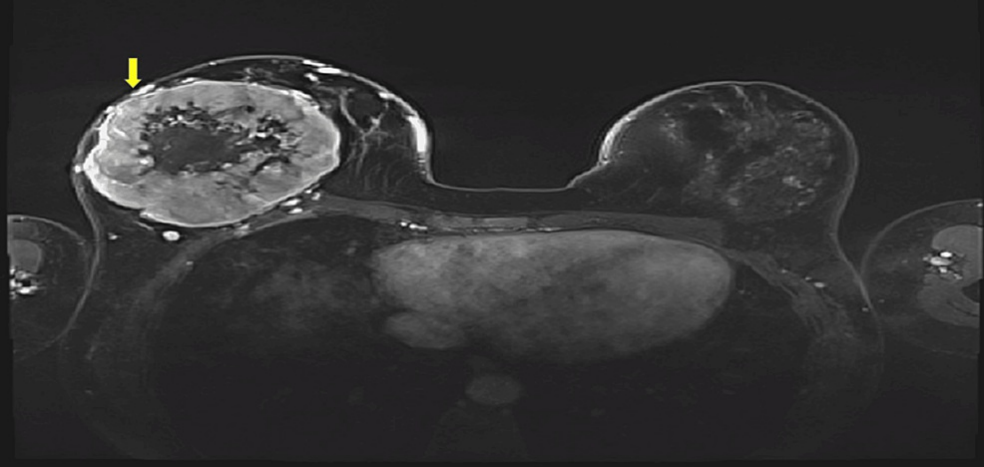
MRI reveals an uneven enhancement pattern and lack of enhancement in the central region.
